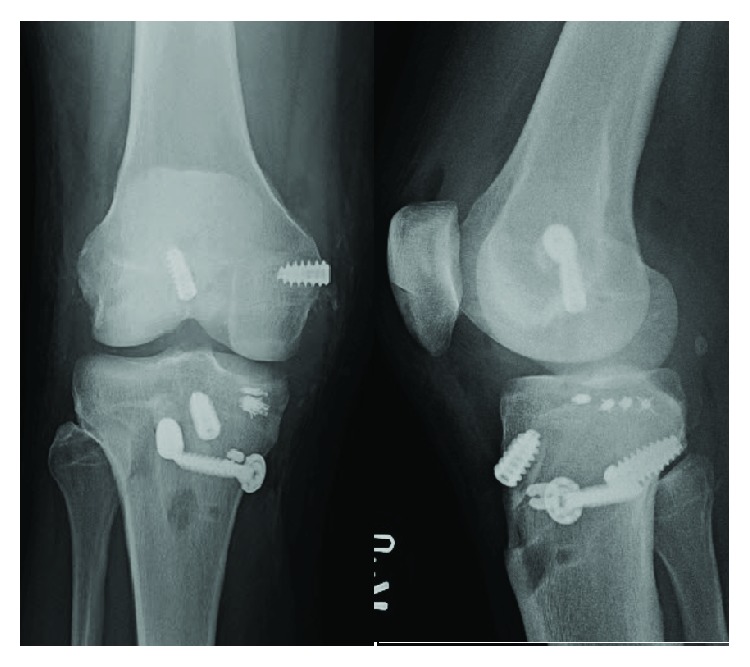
A postoperative anteroposterior and lateral radiograph of the patient's right knee following ACL reconstruction with a bone-tendon-bone autograft, PCL reconstruction with an allograft, MCL repair with additional allograft reconstruction, posterolateral corner reconstruction with an allograft, and repair of the posterior horn of the medial meniscus.
